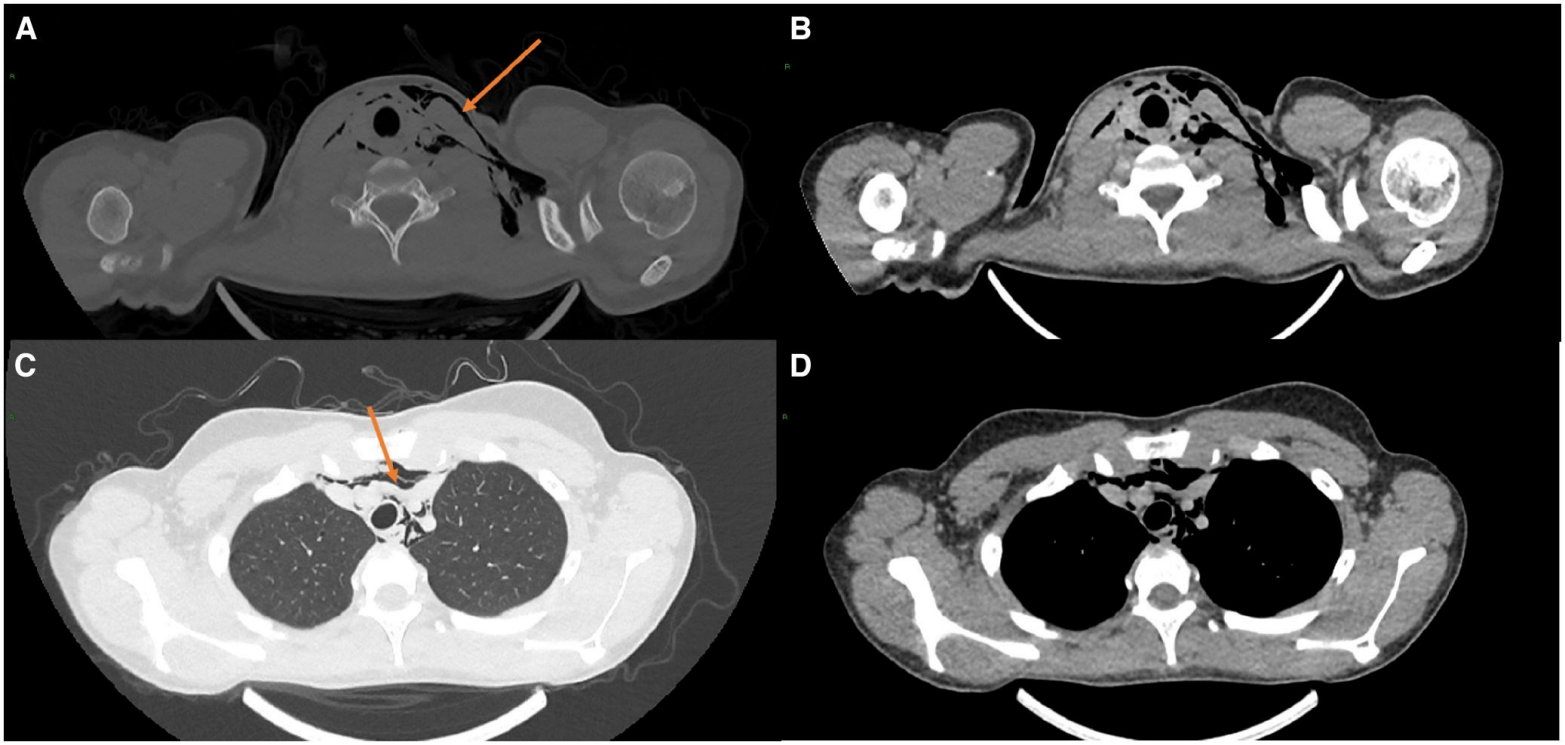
Non-contrast CT thorax: (A) Surgical emphysema predominantly noted in the left neck on bone windows (arrow). (B) Otherwise normal tissue appearances seen on soft tissue windows of the same slice. (C) Mediastinal emphysema evident more inferiorly (arrow) with normal appearance of the lung apices on lung windows. (D) Soft tissue windows of the same slice with otherwise normal soft tissue appearances.
